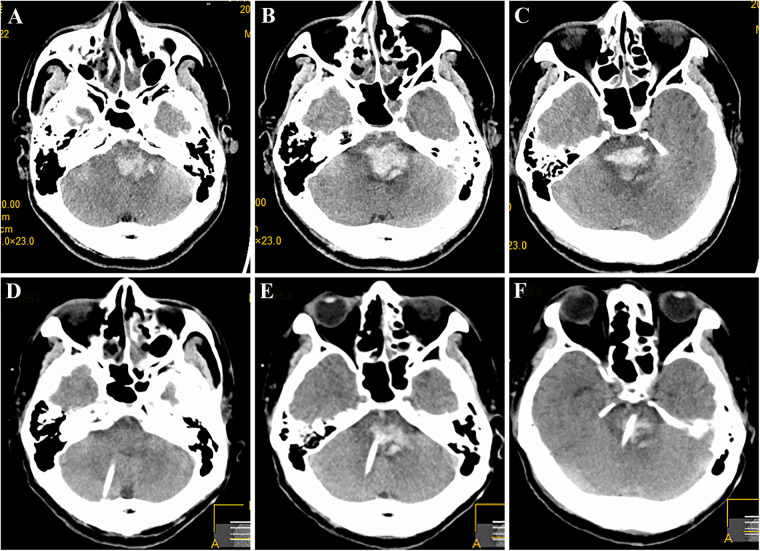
Stereotactic hematoma puncture and drainage with urokinase instillation for hypertensive pontine hemorrhage. (A-C) Computed tomography (CT) of the head demonstrated a pontine hematoma measuring approximately 9 mL in volume, without evidence of hydrocephalus. Emergency stereotactic puncture and drainage of the hematoma were performed. Intraoperatively, approximately 3 mL of altered blood was aspirated. Postoperatively, urokinase (30,000 IU in 2 mL normal saline) was instilled into the hematoma cavity daily via the catheter. (D-F) Follow-up head CT on postoperative day 3 revealed reduction of the hematoma volume to 2 mL.
